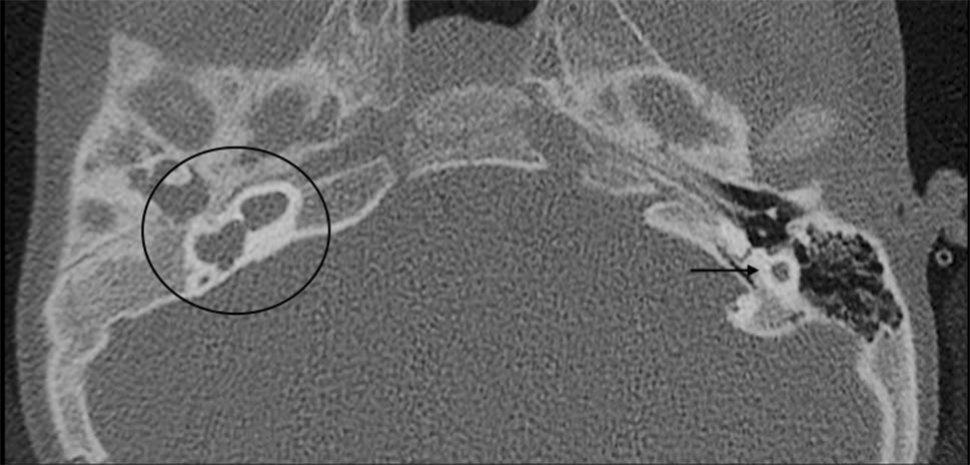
A 2-year-old male with bilateral SN deafness from birth. Axial CT obtained at IAC level shows bilateral atresia of IAC. On the left side the IE structures are absent; a residual otocyst is appreciable (arrow). Formation of the petrous bone is normal but the otic capsule is hypoplastic. On the right side an incomplete partition malformation is also evident (circled)
